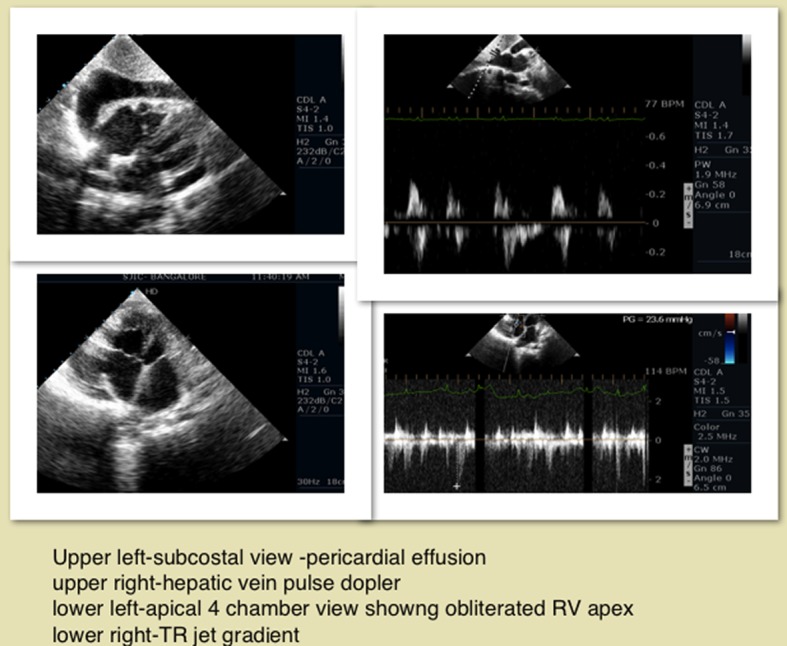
Two-dimensional echocardiogram apical four-chamber, subcostal views showing obliterated RV apex, pericardial effusion, hepatic vein respiratory variation, TR jet.
